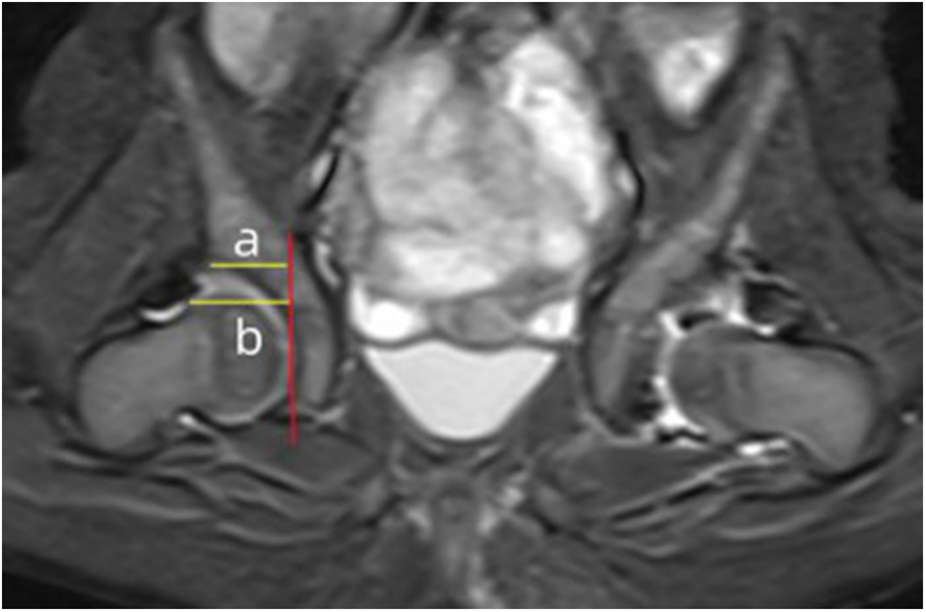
Evaluation of the CAHI. On the MRI coronal image showing the maximum diameter of the femoral head, a vertical line was drawn on the innermost edge of the femoral head cartilage. The vertical distance between the line and the outermost edge of the acetabular cartilage (a) and the distance between the line and the outermost edge of the femoral head cartilage (b) were measured. The percentage of these two distances is the CAHI (CAHI = a/b × 100).
